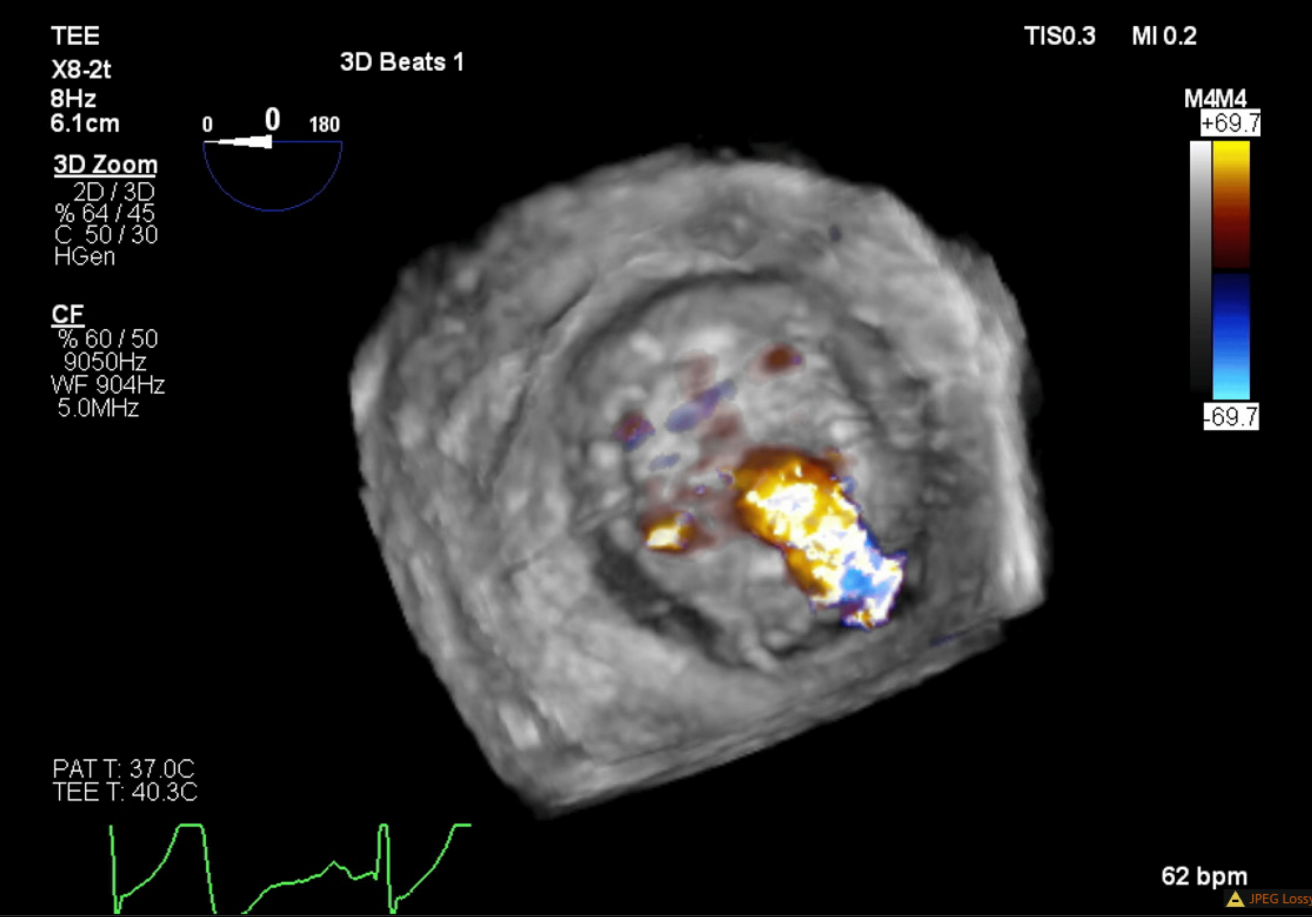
Transesophageal echocardiogram three-dimensional reconstruction with color Doppler, demonstrating paravalvular regurgitation originating from the posterior aspect of the mitral valve prosthesis.
